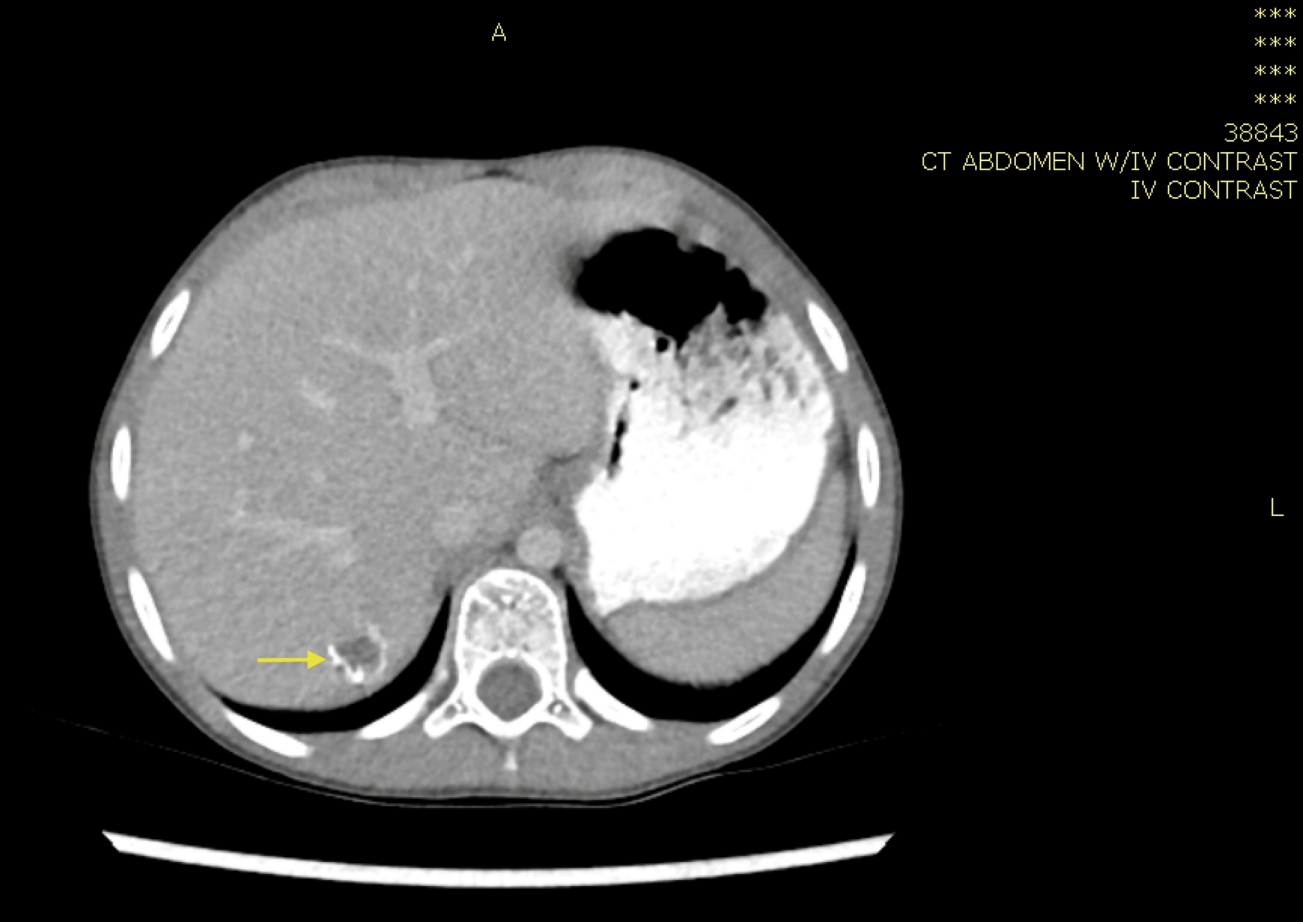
Computed tomography of the abdomen showing liver with normal size and homogenous enhancement with no obvious underlying parenchymal disease. Significant circumferential wall thickening was observed involving the ascending colon (yellow arrow), hepatic flexure, and mesentery lymphadenopathy, with impression of basidiobolomycosis fungal infection with right-sided bowel wall thickening and hepatic abscess formation.
